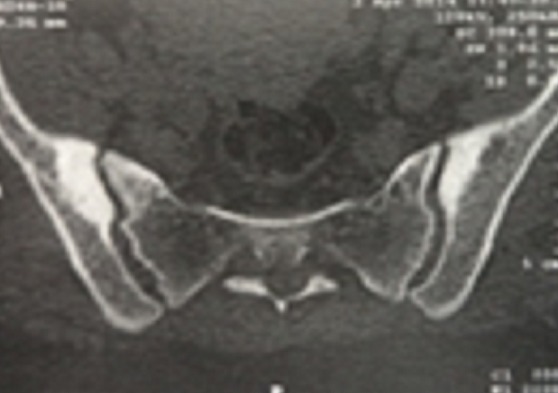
CT scan showing condensation of the iliac sides of sacro iliac joints
